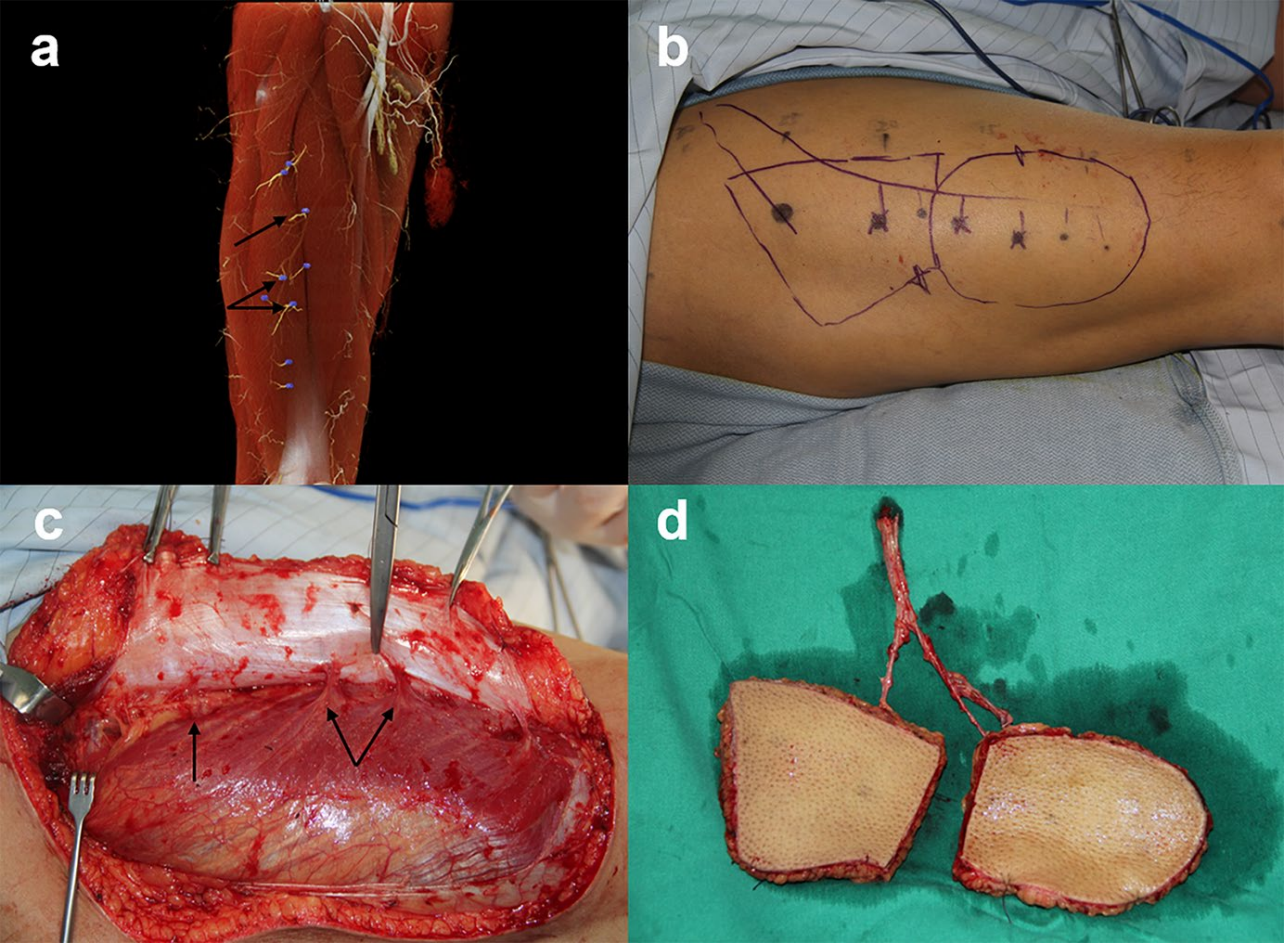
A case example of lobed flap harvesting guided by preoperative CTA mapping. a–b CTA mapping showed the location of perforators and guided the design of a lobed flap. c–d Perforators observed during the operation were consistent with the preoperative CTA assessment, and the lobed flap was successfully obtained
